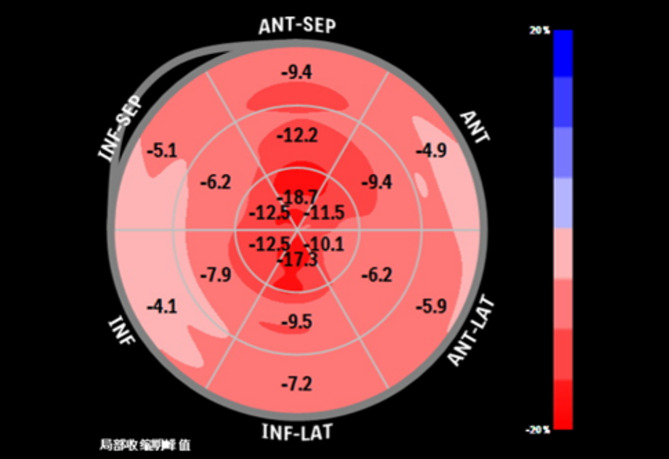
Speckle tracking echocardiography. Spect-tracking echocardiography indicated left ventricular enlargement, weakened left ventricular wall diffuse motion, enhanced left ventricular myocardial echo, local calcification of aortic valve, mild regurgitation of mitral and tricuspid valves, simEF: 27%, and a small amount of pericardial effusion
